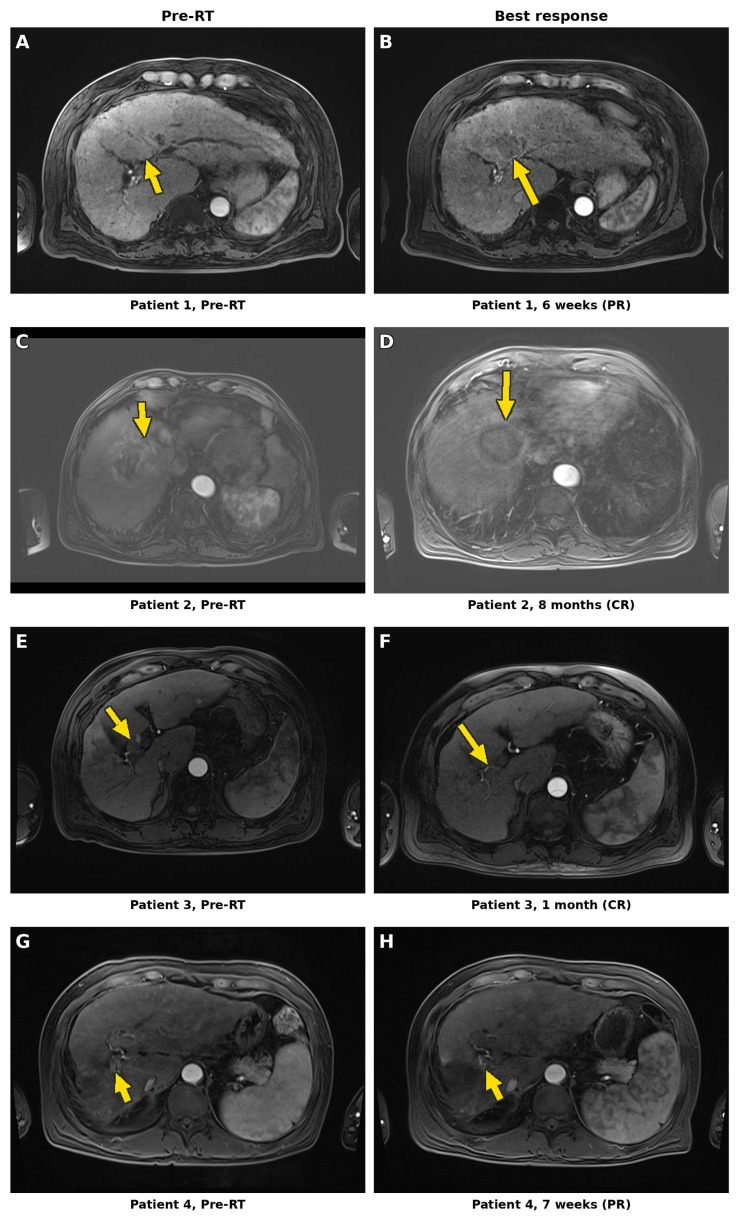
For each patient, the left panel shows contrast-enhanced arterial-phase MRI at baseline (pre-RT) and the right panel shows contrast-enhanced arterial-phase MRI at the time of best response. Yellow arrows indicate the bile duct tumor thrombus. Patients are presented in chronological order of treatment. (A,B) Patient 1. Pre-RT MRI demonstrating the left bile duct tumor thrombus extending to the hilum with the contiguous S4 mass (3.0 cm) and obstructive jaundice. Post-RT MRI at six weeks showing partial response of the irradiated target with resolution of biliary obstruction. (C,D) Patient 2. Pre-RT MRI demonstrating the intraductal thrombus within the segment 8 intrahepatic bile duct. The S8 mass (7.2 cm) and S4 mass (2.7 cm) were excluded from the primary target volume. Post-RT imaging at eight months confirming complete response of the irradiated thrombus as well as both non-targeted hepatic masses. Loss of arterial enhancement in all three lesions was already evident on CT performed one week after treatment completion. (E,F) Patient 3. Pre-RT MRI demonstrating the S5/8 mass (2.5 cm) with right bile duct tumor thrombus. Post-RT MRI at one month showing complete response of the irradiated target with resolution of intrahepatic ductal dilatation. (G,H) Patient 4. Pre-RT MRI demonstrating the large S4 and right lobe mass with right intrahepatic duct invasion and involvement of the left hepatic duct, and concurrent right portal vein tumor thrombosis. Post-RT MRI at seven weeks showing partial response. A reduced dose of 40 Gy in 5 fractions was prescribed owing to the large target volume and concurrent right portal vein tumor thrombosis. RT, radiotherapy; CR, complete response; PR, partial response.
